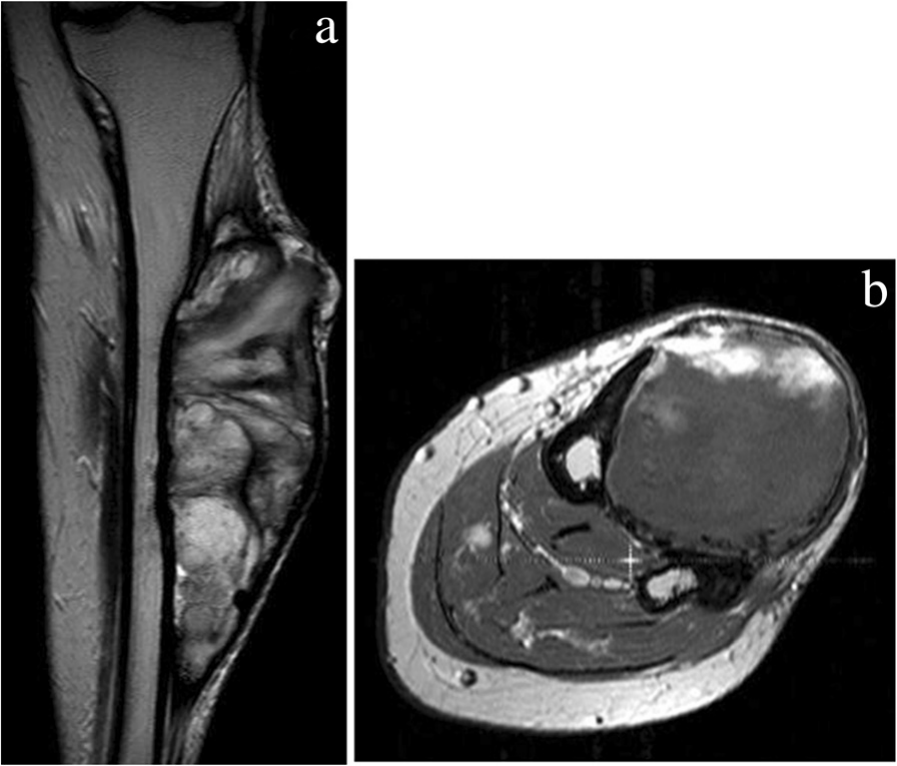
One-year follow-up magnetic resonance imaging. (a) Coronal T2-weighted, (b) axial T1-weighted fat-suppressed postgadolinium contrast enhancement.
